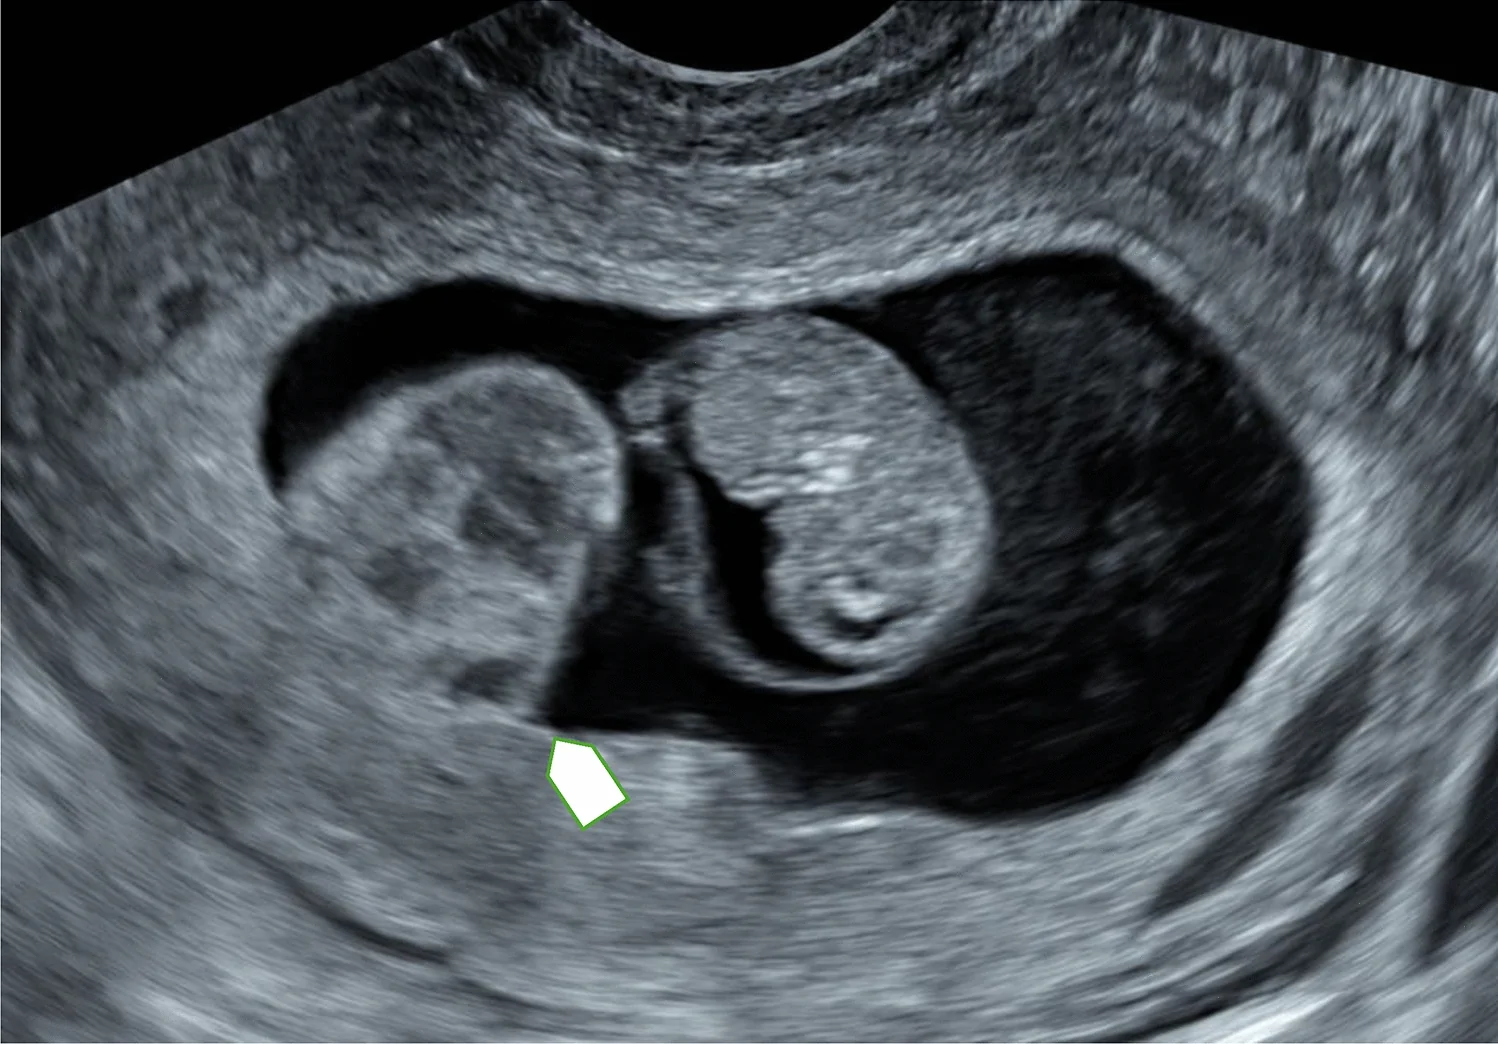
Chorionic bump (arrow) seen protruding into gestational sac next to a viable foetus on transvaginal ultrasound scan
